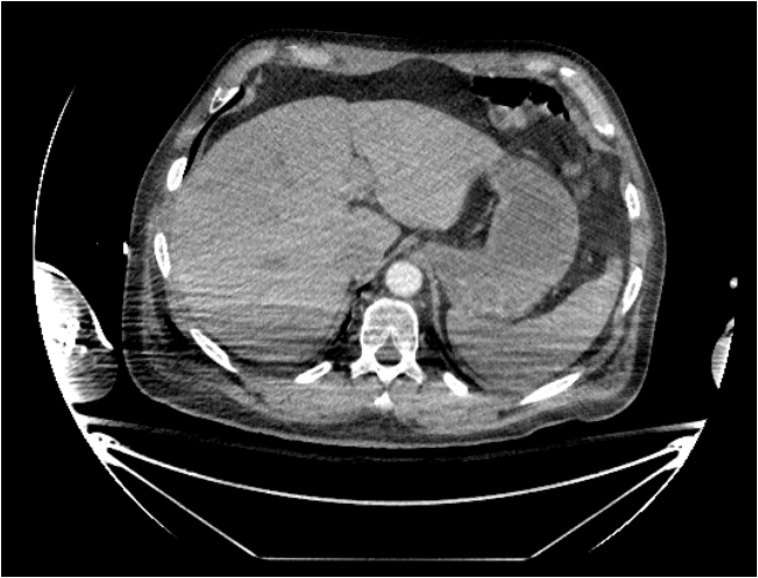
An axial view/cross-section of a CT scan of the abdomen reveals a normal appearance of the spleen with no evidence of splenic laceration on index imaging of the spleen.
